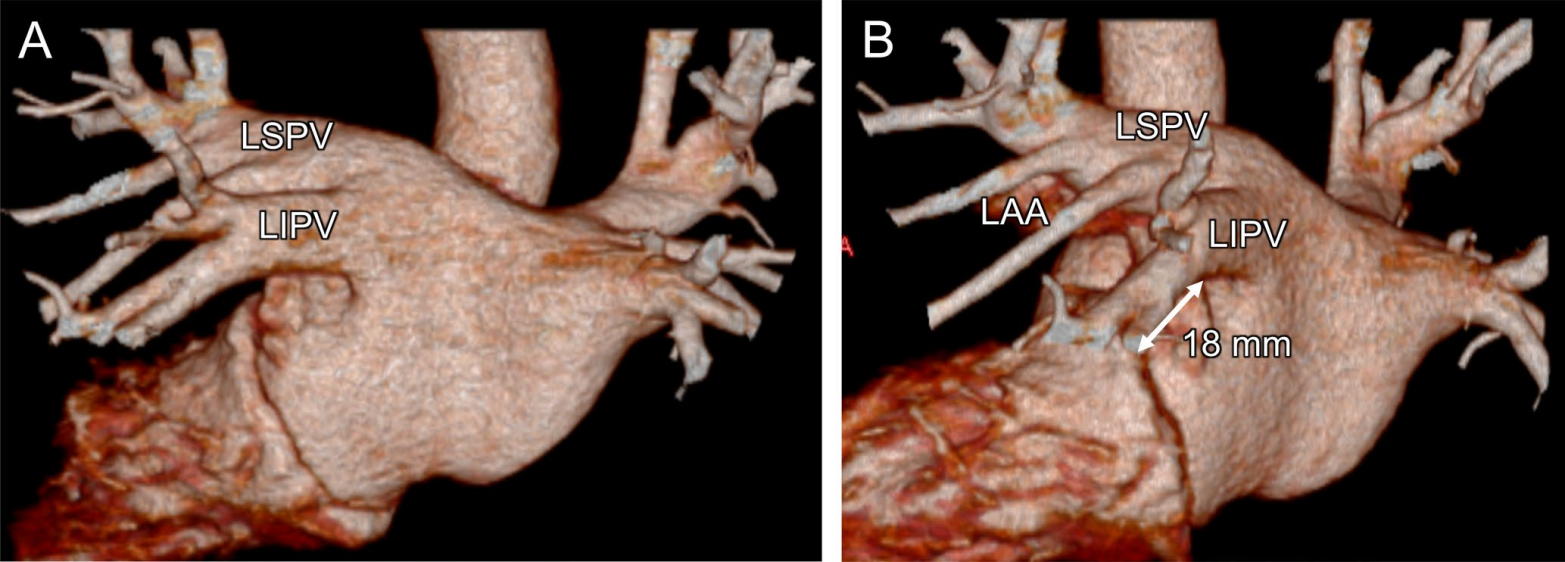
Three‐dimensional computed tomography of the pulmonary veins before the procedure. (A) Posterior–anterior view. (B) Left posterior oblique view. The white arrow indicates the mitral isthmus, which measures approximately 18 mm in length. LSPV, left superior pulmonary vein; LIPV, left inferior pulmonary vein; LAA, left atrial appendage.
